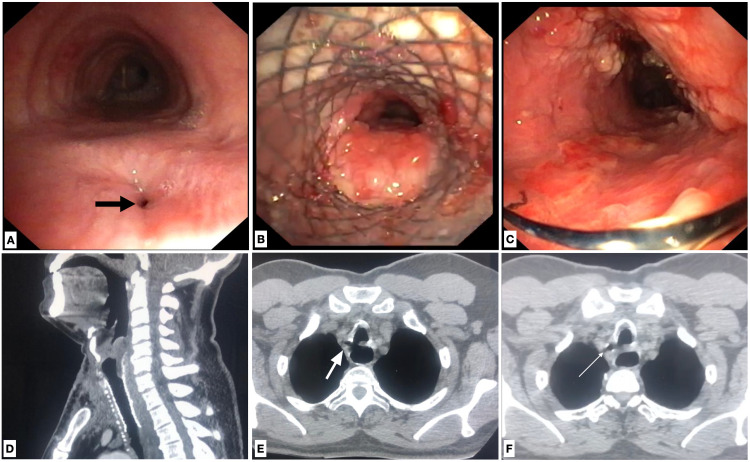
(A) Bronchoscopy showing the fistula (block arrow) in the membranous part of the trachea, (B) tracheal SEMS with granulation tissue, (C) post-stent removal granulation tissue in the trachea, (D) CT demonstrating the SEMS placement, (E) persistence of the fistula (block arrow) post-removal of SEMS, and (F) air foci in the tracheo-esophageal groove (line arrow) post-removal of SEMS. SEMS: self-expandable metallic stents.
